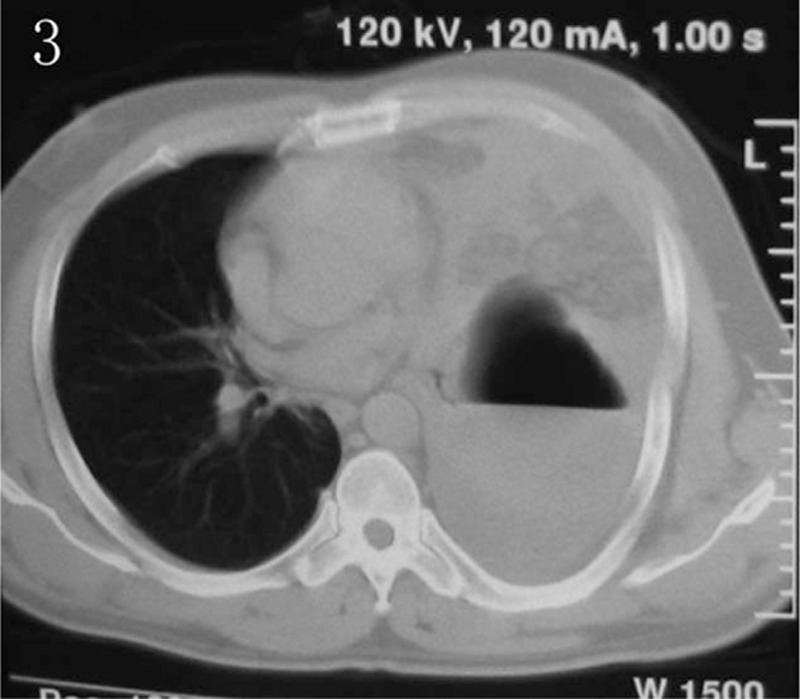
Computed tomography (CT) scan indicating the stomach and bowel loops herniated into the left thorax.
